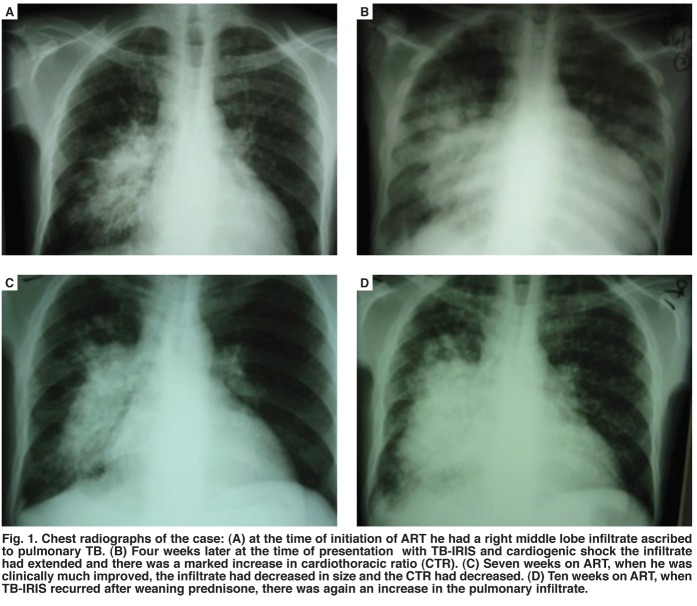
Chest radiographs of the case: (A) at the time of initiation of ART he had a right middle lobe infiltrate ascribed to pulmonary TB. (B) Four weeks later at the time of presentation with TB-IRIS and cardiogenic shock the infiltrate had extended and there was a marked increase in cardiothoracic ratio (CTR). (C) Seven weeks on ART, when he was clinically much improved, the infiltrate had decreased in size and the CTR had decreased. (D) Ten weeks on ART, when TB-IRIS recurred after weaning prednisone, there was again an increase in the pulmonary infiltrate.
